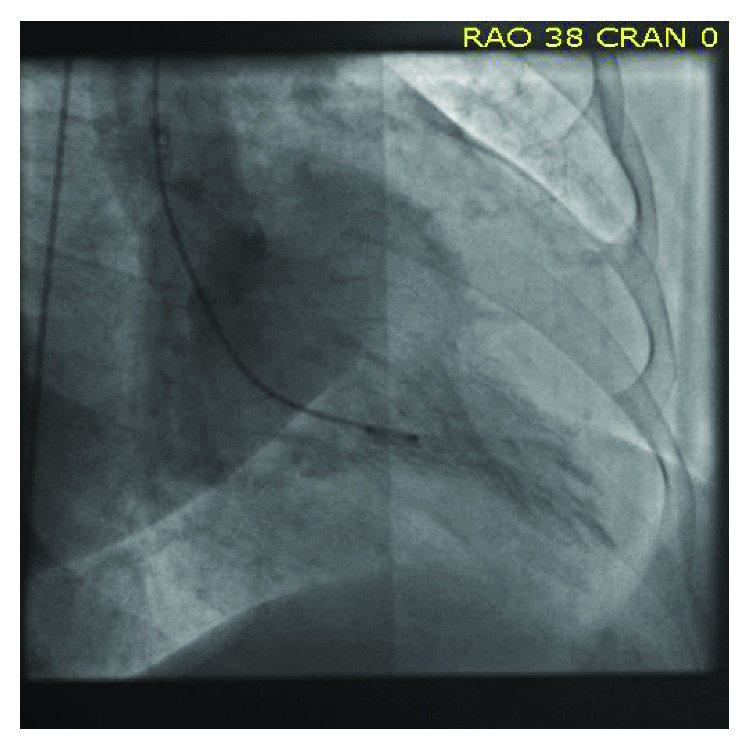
Cardiac left ventriculogram done by cardiac chamber catheterization confirming the echocardiographic finding of apical hypertrophy.
